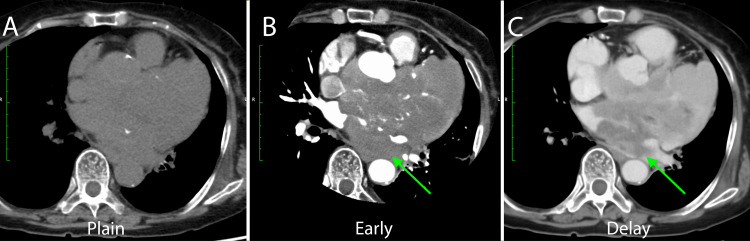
Findings on CT scans. (A) Plain CT. (B) Early-enhanced CT showed feeding arteries to the tumor (green arrow). (C) Delay enhanced indicated a low enhanced area of the cardiac tumor (green arrow). These features suggested that the tumor was rich in blood vessels.
